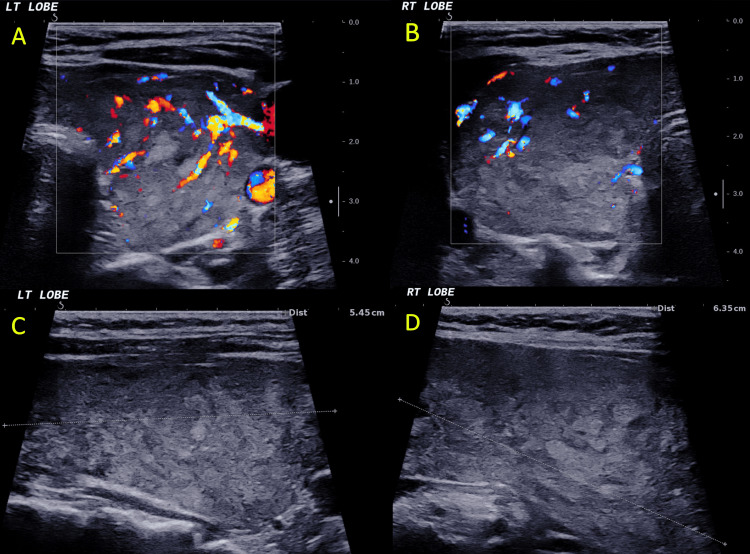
Thyroid ultrasonography with color Doppler demonstrating diffuse hypervascularity consistent with Graves’ disease. (A–B) Color Doppler images of the left (LT) and right (RT) thyroid lobes showing diffusely increased intraparenchymal vascularity (“thyroid inferno” pattern), supportive of Graves’ disease. (C–D) Corresponding grayscale (B-mode) longitudinal views demonstrating mild diffuse thyroid enlargement with a heterogeneous echotexture (longitudinal measurements: LT lobe ~5.45 cm, RT lobe ~6.35 cm).
